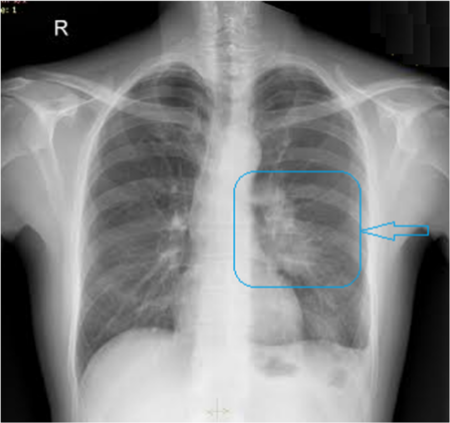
Chest X-ray showed obliteration of the left costophrenic angle and increased inhomogenous density in the left parahilar zone.
